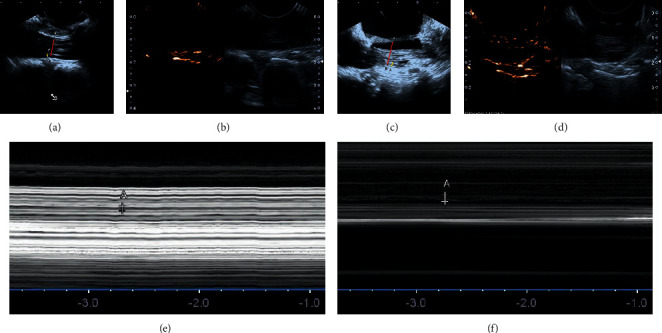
Intraoperative ultrasonography (IoUS) of the case: (a) preoperative diameter of the L5 nerve root (yellow line) and dura mater (red line); (b) preoperative blood perfusion of the L5 nerve root (white arrow); (c) postoperative diameter of the L5 nerve root (yellow line) and dura mater (red line); (d) postoperative blood perfusion of the L5 nerve root (white arrow); (e) preoperative amplitude of the L5 nerve root; and (f) postoperative amplitude of the L5 nerve root.
